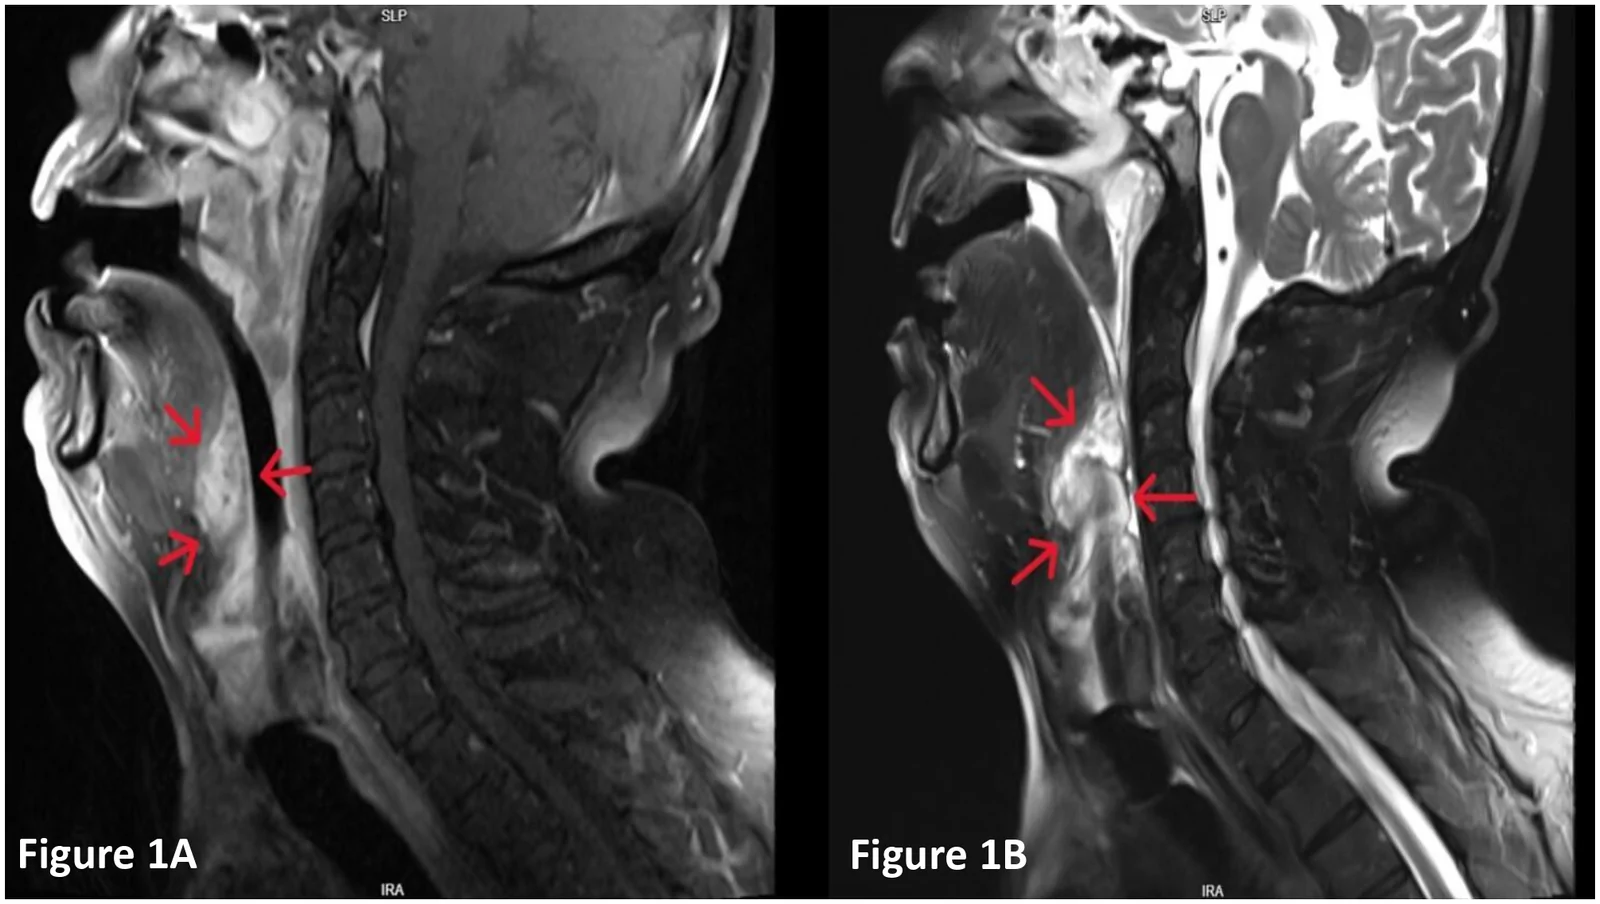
Contrast-enhanced neck MRI. (A) T1-weighted image showing hypointensity with diffusion restriction, heterogeneous enhancement, and focal areas of necrosis. (B) T2-weighted image showing a left supraglottic mass with heterogeneous hyperintensity.
